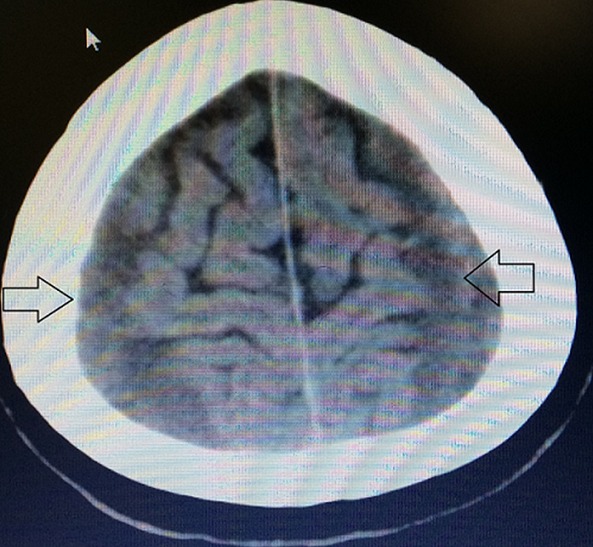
Initial cranial CT scans show a 20 mm thick low-isodensity chronic subdural hematoma at bilateral frontotemporoparietal region
